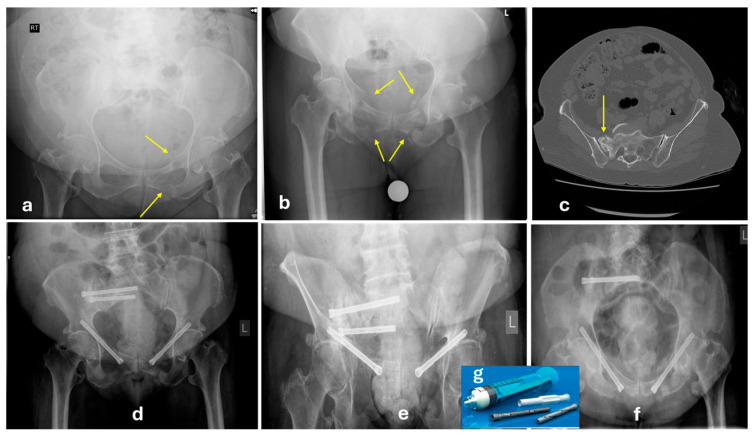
Case example of a 71-year-old female patient nursing home resident with a history of dementia, obesity, and rheumatoid arthritis. (a) Initial anteroposterior plain X-ray following a low-energy fall demonstrating fractures of the left superior and inferior rami (yellow arrows). Without further imaging (and with the limitations of the clinical examination due to her cognitive status and prior low back pain), she was diagnosed with an FFP-Ia stable unilateral anterior fracture and allowed to mobilise freely. No appropriate follow-up was organised, and the patient was discharged back to the nursing home. (b) Subsequent plain anteroposterior X-ray 8 months later, demonstrating fractures of all four rami (yellow arrows), incomplete healing of the initial left fractures, whilst she is now bed-bound and refusing to get out of bed due to pain. (c) Axial cut of a CT-scan with a Denis 2 type of sacral fracture with evidence of chronicity and nonunion (FFP-IIIc). (d) Anteroposterior (e) outlet, (f) inlet plain X-rays of the pelvis 3 months post-operatively following percutaneous fixation with bilateral retropubic fenestrated cannulated screws and left iliosacral screws at S1 and S2 levels augmented with calcium phosphate cement (g). The patient at this stage was mobilising unaided without complaining of pain.
